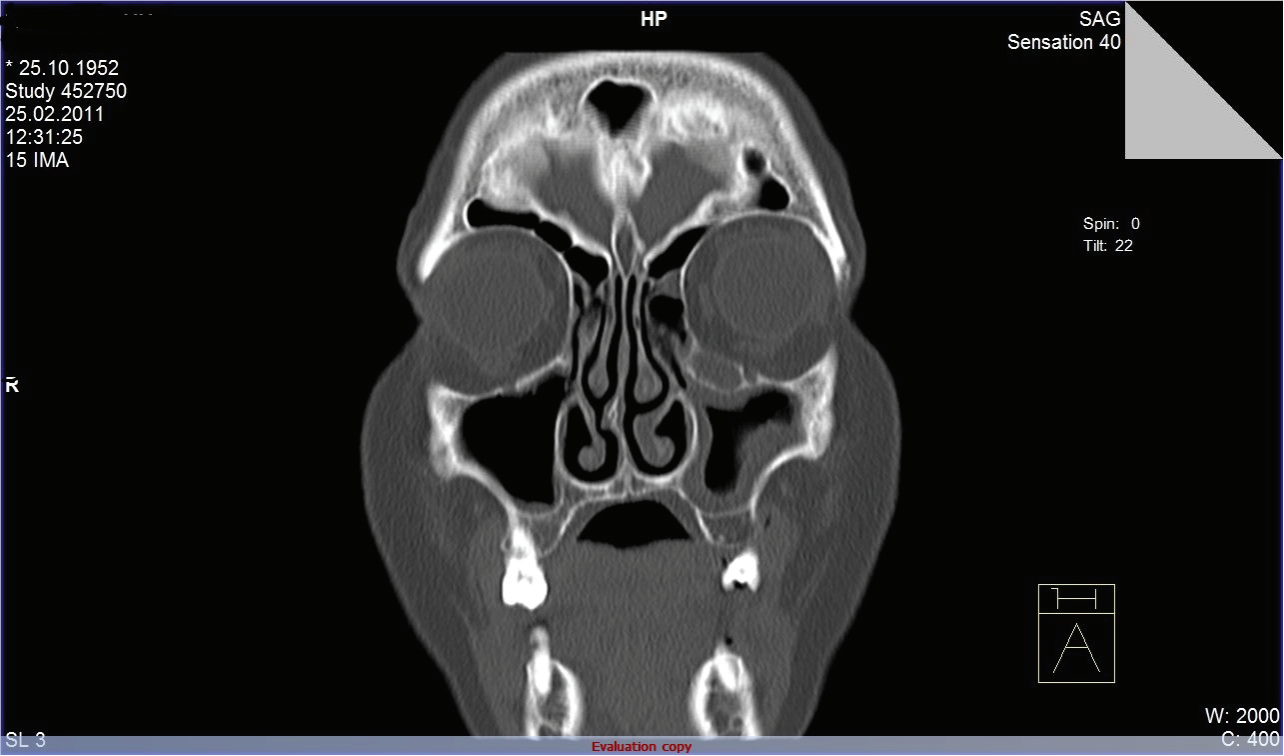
Paranasal sinus computed tomography scan. It shows clear chronic sinusitis.
